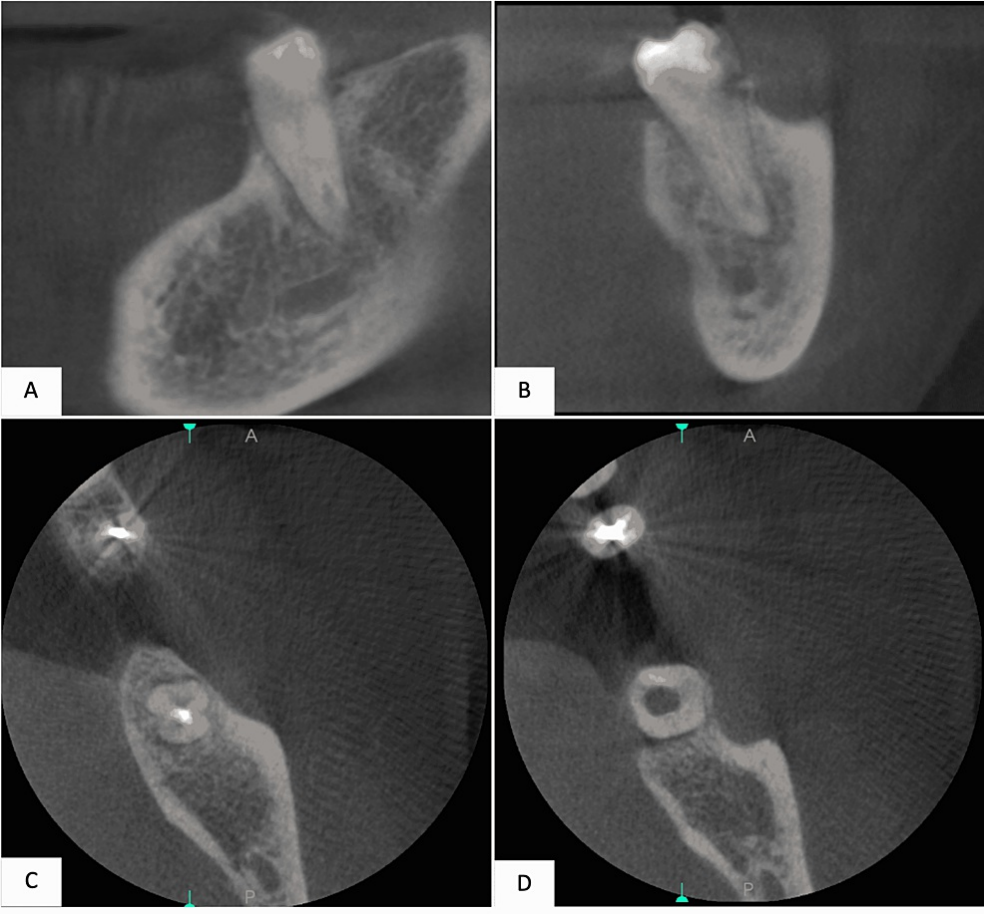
Figure 3: Cone-beam computed tomography (CBCT) for tooth #37 taken at 1 mm slice thickness. Images A and B: Coronal slicing Images C and D: Axial slicing
